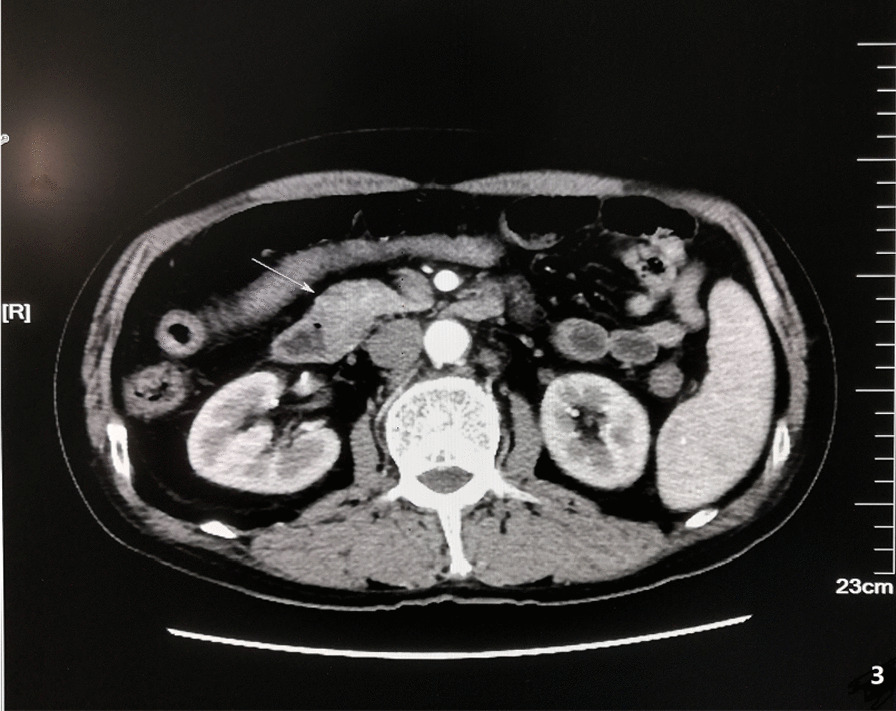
Abdominal enhancement CT: duodenal papilla space-occupying lesions, intrahepatic and extrahepatic bile duct dilatation
